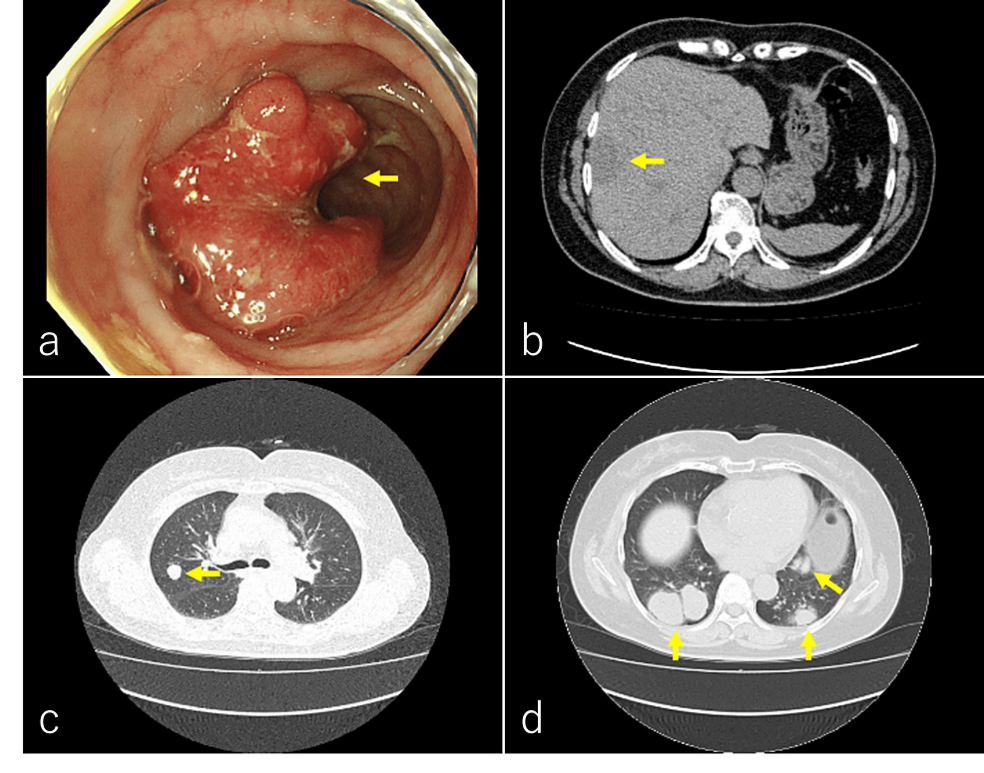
Lower endoscopy in the sigmoid colon and axial CT. Lower endoscopy revealed an elevated lesion in the sigmoid colon (a, yellow arrow). Abdominal CT showed hypodense lesions in the liver (b, yellow arrow). Lung CT showed multiple nodules in the bilateral lungs (c and d, yellow arrows).
